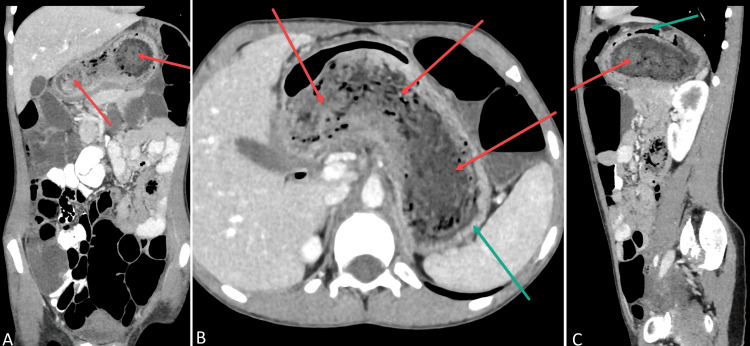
Computed tomography images - coronal (A), axial (B) and sagittal (C) views of abdomen showing stomach bezoar (red arrows) extending through entire stomach (green arrows).
